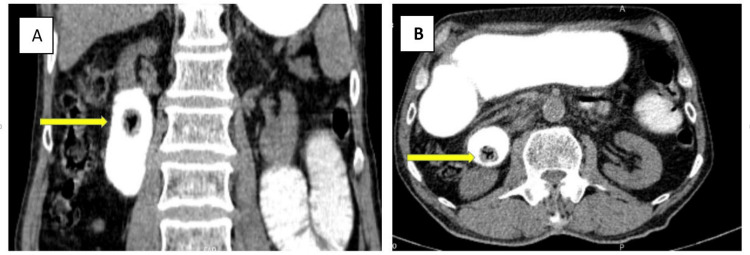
Oral contrast-enhanced CT - 2 The images revealed a recent duodenal ectopic non-obstructing gallbladder stone with a typical Mercedes-Benz sign (A, B) CT: computed tomography
